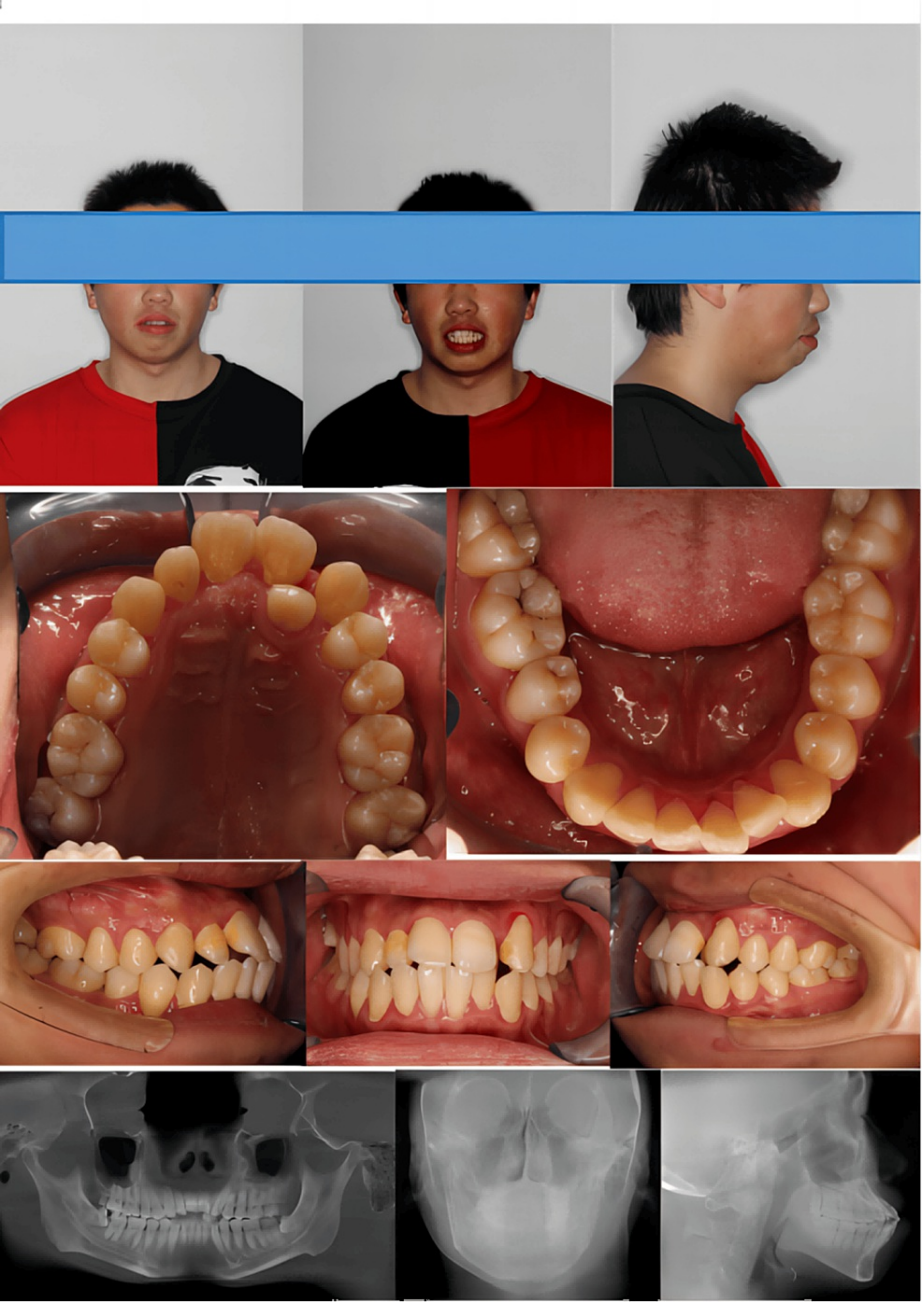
Pretreatment facial and intraoral photographs, lateral cephalogram, and panoramic radiograph
